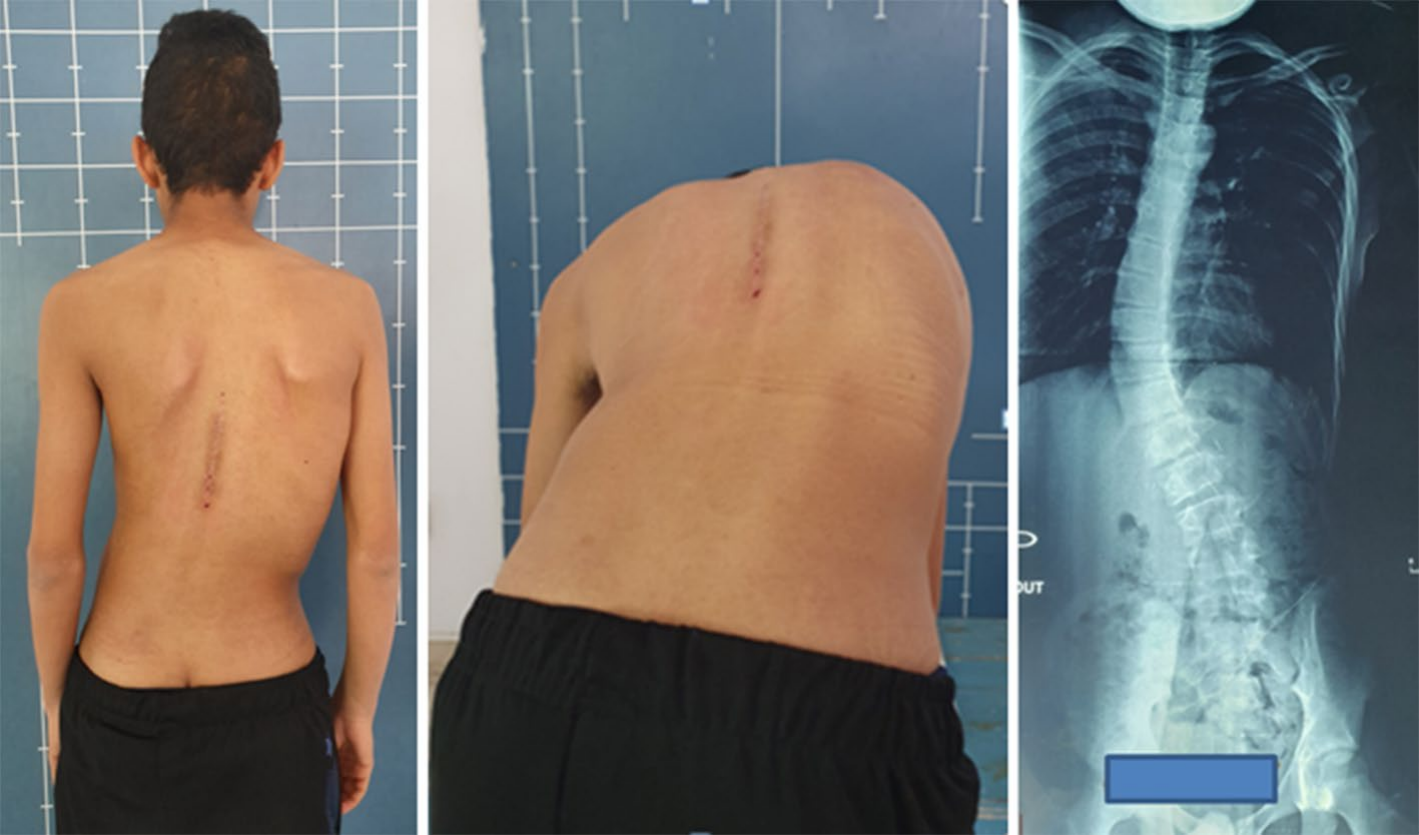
In standing position: deformation of the midline, leaning forward: straight gibbosity and the x-ray shows structural scoliosis
